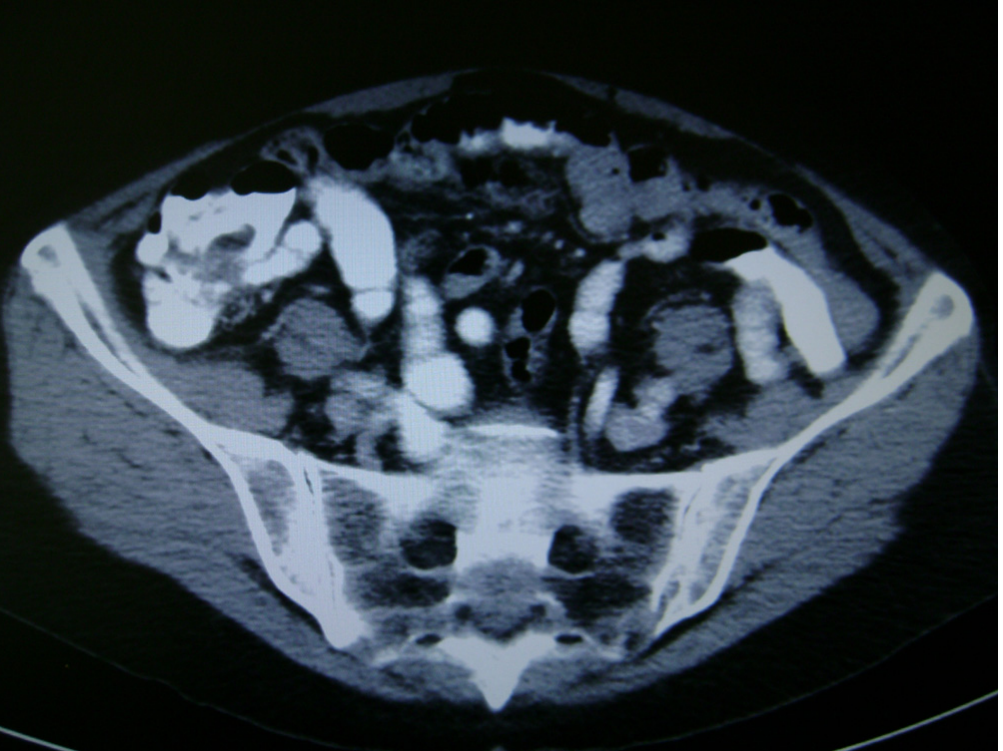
Pre-operative CT Abdomen Pelvis image showing normal appearing ileum at ileo-caecal junction.
